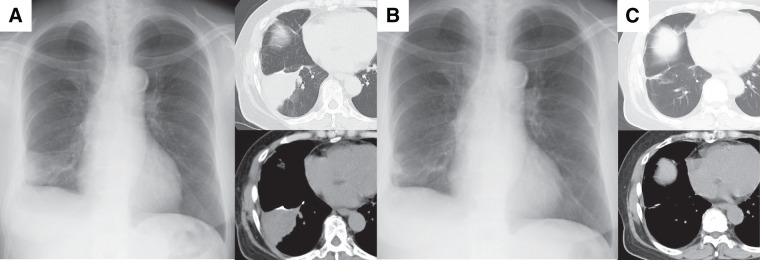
Postoperative CT images of Case 1. A mass adjacent to the staple line was found on the postoperative CT scan and chest X-ray, which were obtained 9 months after surgery (A). A chest X-ray, which was performed 1 month after administration of tranilast (B); it showed that the mass shrank quickly in size; by the 3rd month after tranilast administration the CT scan showed that the mass had disappeared (C).
